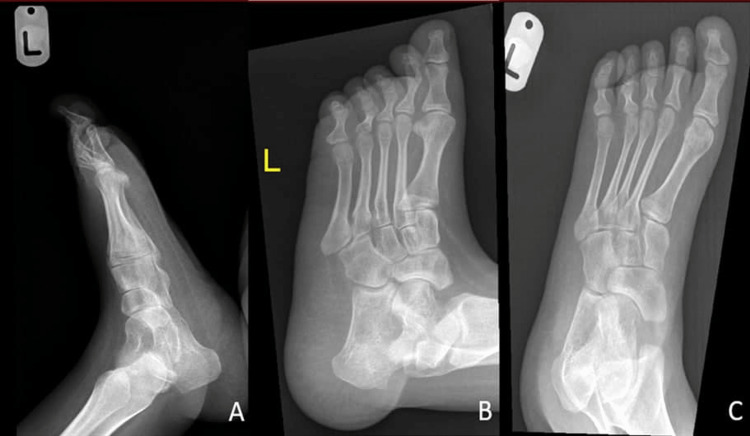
Preoperative X-ray of the left foot: (A) lateral, (B) oblique, and (C) anteroposterior views
